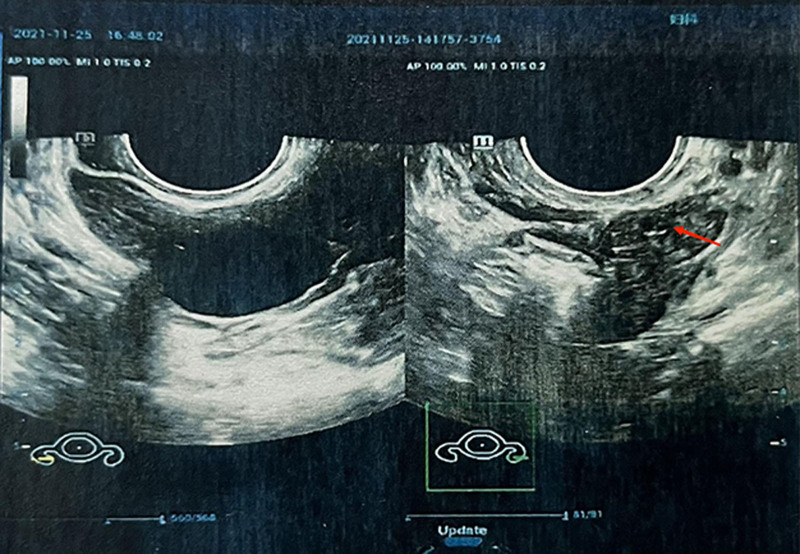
(November 2021) Transvaginal ultrasound showed 1 antral follicle count (AFC) observed in the left ovary.
